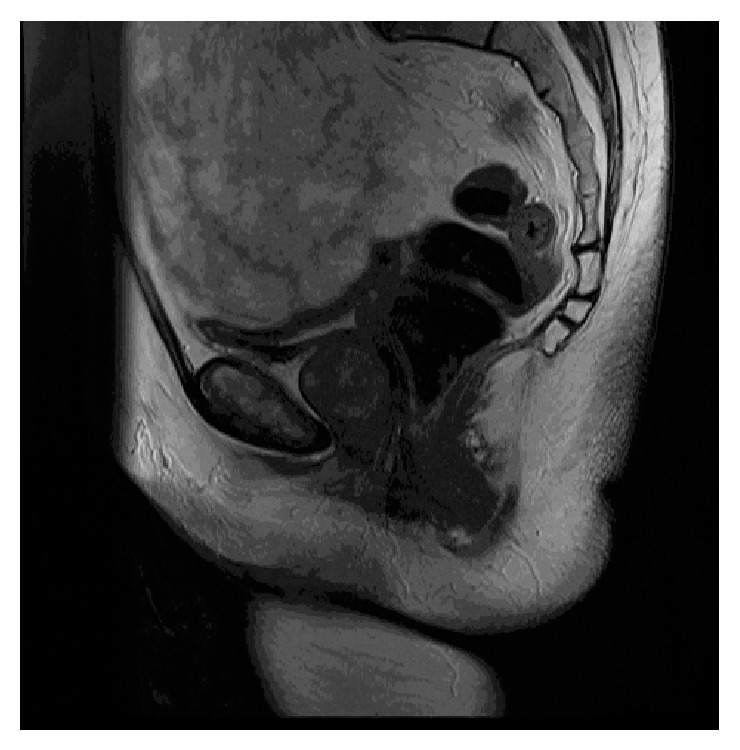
MRI of paraurethral leiomyoma in relation to urethra.
